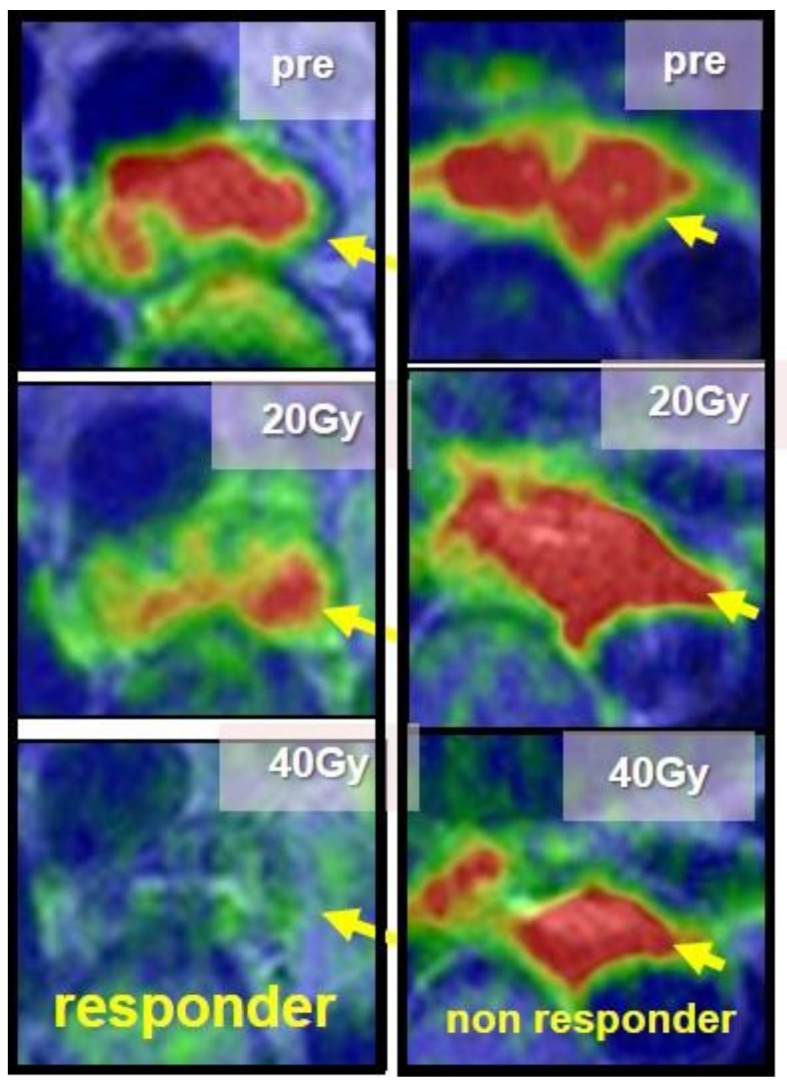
Comparison of the change in diffusion-MRI images of the tumor over the course of CRT for esophageal squamous cell carcinoma between responders and non-responders. Arrows indicate the tumor. Responders showed higher change in tumor diffusion than non-responders.
